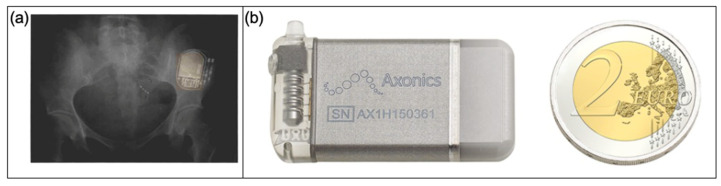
Nerve stimulation devices. (a) An anterior–posterior radiograph showing the InterStim device in upper buttock, with a lead electrode in sacral vertebrae (S4) foramen [40]; (b) the Axonics neurostimulator device next to a coin [84]. Copyright 2018, John Wiley and Sons.
